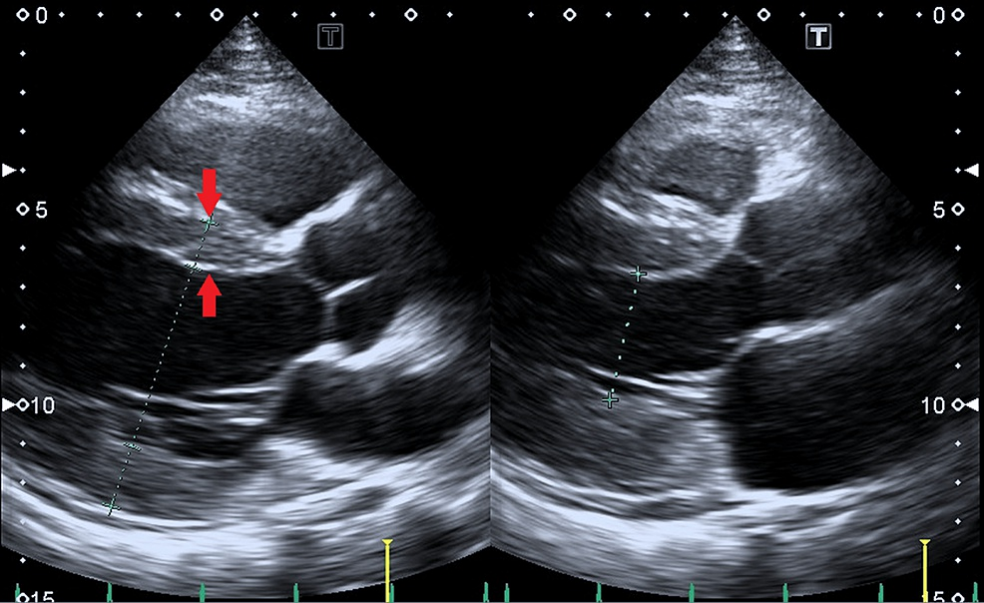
Echocardiogram on hospital admission in case 2. The left and right panels indicate diastolic and systolic phases of parasternal long-axis views, respectively. Preserved left ventricular ejection fraction of 59% and concentric left ventricular hypertrophy with interventricular septum thickness of 13 mm (red arrows) were noted.
